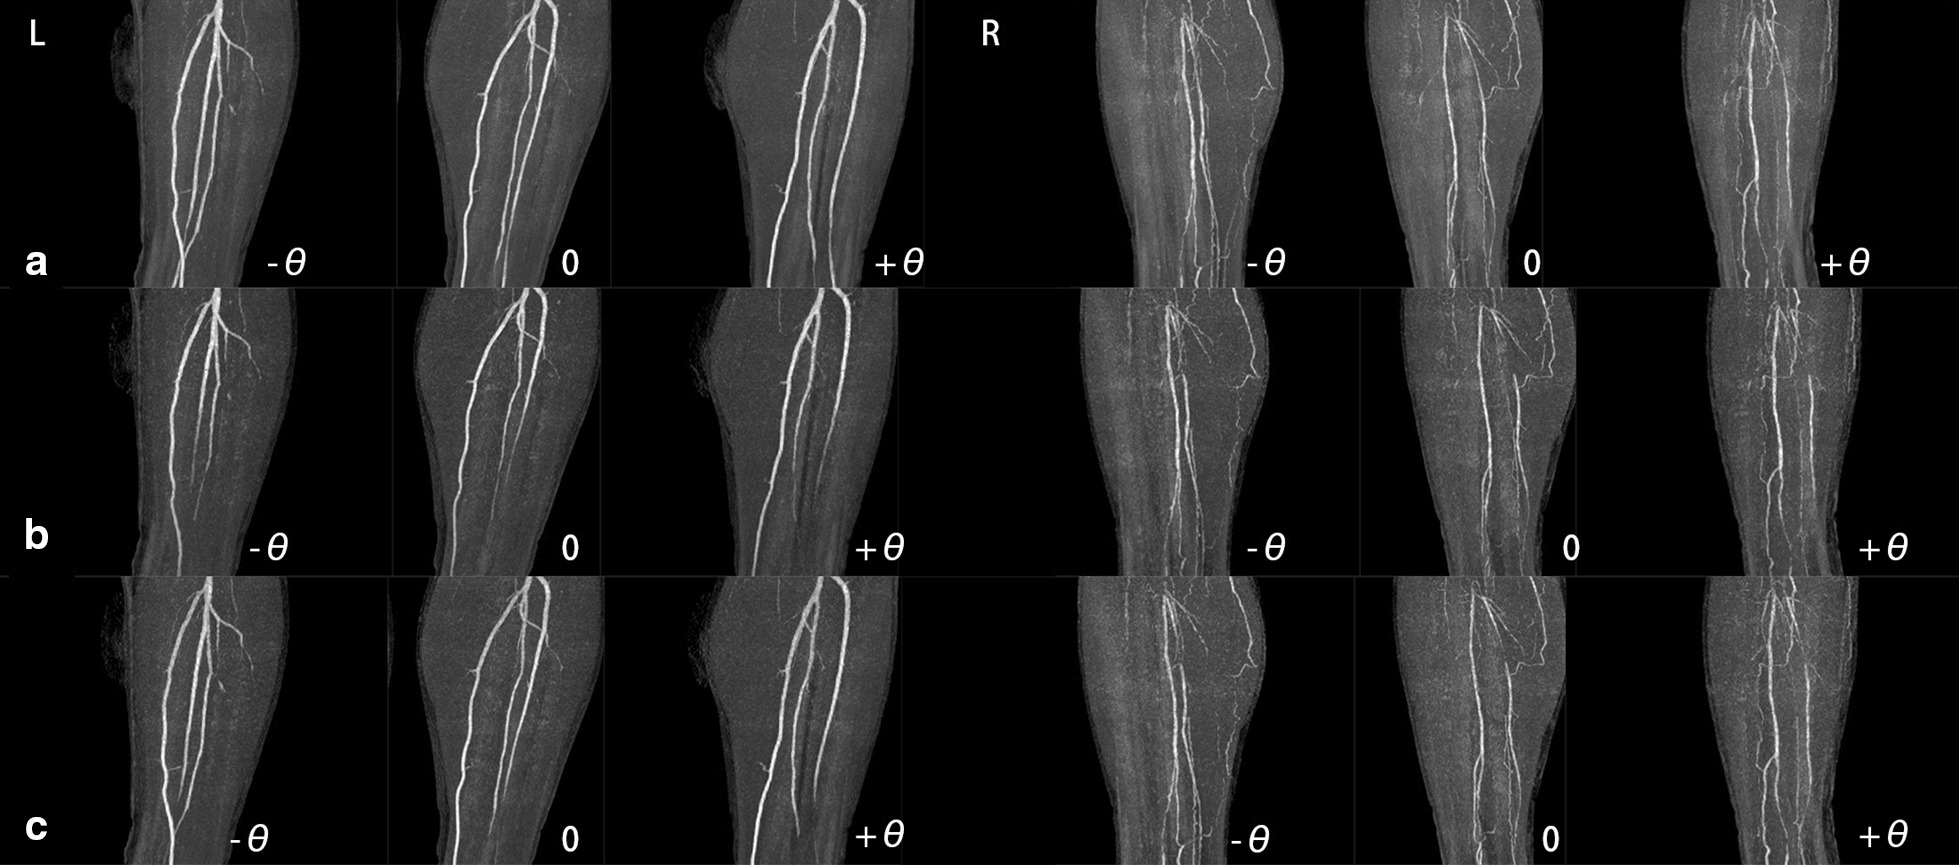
Comparison of t-MIP CTA and different phases of dyn-CTA of the lower extremities for a male patient with ischemia of the right lower extremity and normal left lower extremity. a The t-MIP images, and the proximal and distal vessel segments of the arteries of the lower legs show optimal enhancement. b and c MIP images of the second and third phases of dyn-CTA. The proximal vessel segments are more enhanced in the second phase, while the distal vessel segments are more enhanced in the third phase. 0, sagittal position; + θ, 45 ° lateral position; −θ, −45° lateral position
